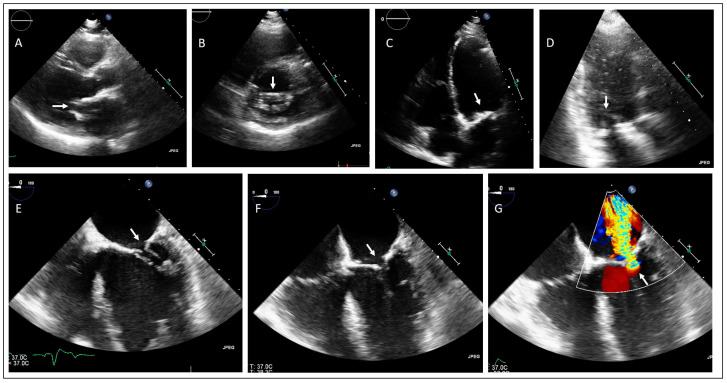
Transthoracic (A–D) and transesophageal (E–G) echocardiographic examinations of the proband of family 1 showing mitral valve stenosis with aortic valve bioprosthesis (Toronto root) in situ. (A) Parasternal long axis view, (B) parasternal short axis view, (C) apical 4-chamber view and (D) apical 3-chamber view, showing severe mitral valve calcification (arrow). (E) Midesophageal 4-chamber view in diastole and (F) systole including (G) color Doppler, showing mitral valve stenosis and regurgitation (arrow).
